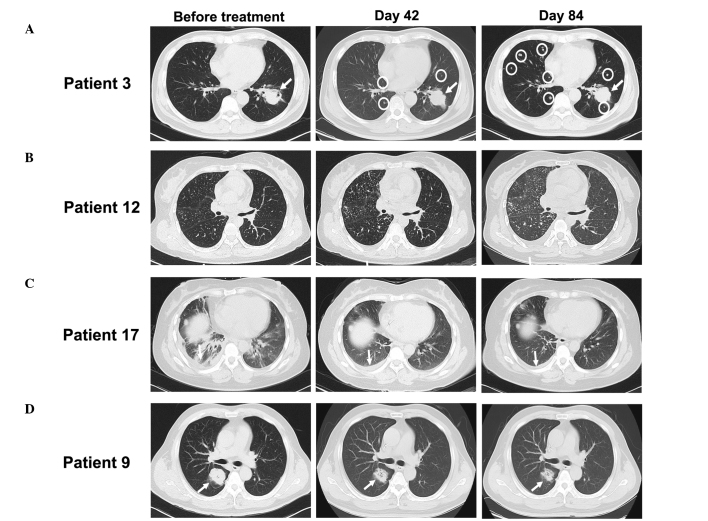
Computed tomography of patients with various responses to cisplatin/pemetrexed chemotherapy. (A) PD in a patient who progressed with an obvious increase in the volume of pulmonary nodules (white arrows) and more emerging micro-lung metastases (white circles). (B) SD in a patient with more emerging micro-lung metastases and pleural effusion (arrows). (C) SD with less pleural effusion (arrows). (D) PR in a patient. The arrows indicate an obvious regression in the volume of pulmonary nodules. Day 42 and day 84 indicate two and four cycles of cisplatin/pemetrexed respectively. PD, progressive disease; SD, stable disease; PR, partial response.
